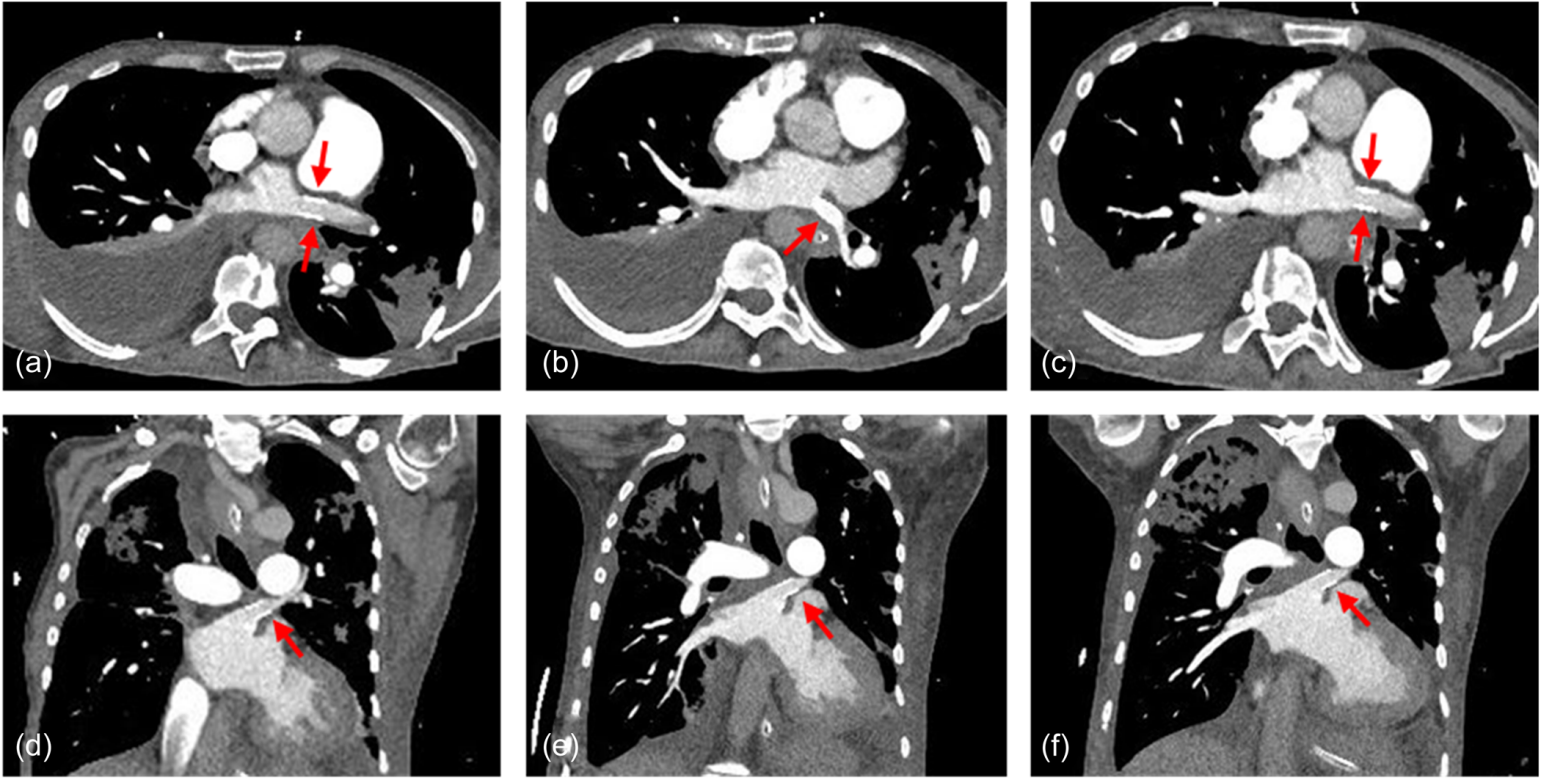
CT angiography at follow‐up examination 4 months after the clinical course. (a, d) Successful stenting of LSPV. (b, e) Successful stenting of LIPV. (c, f) Successful balloon dilatation of RIPV. CT, computed tomography; LIPV, left inferior pulmonary vein; LSPV, left superior pulmonary vein; RIPV, right inferior pulmonary vein.
